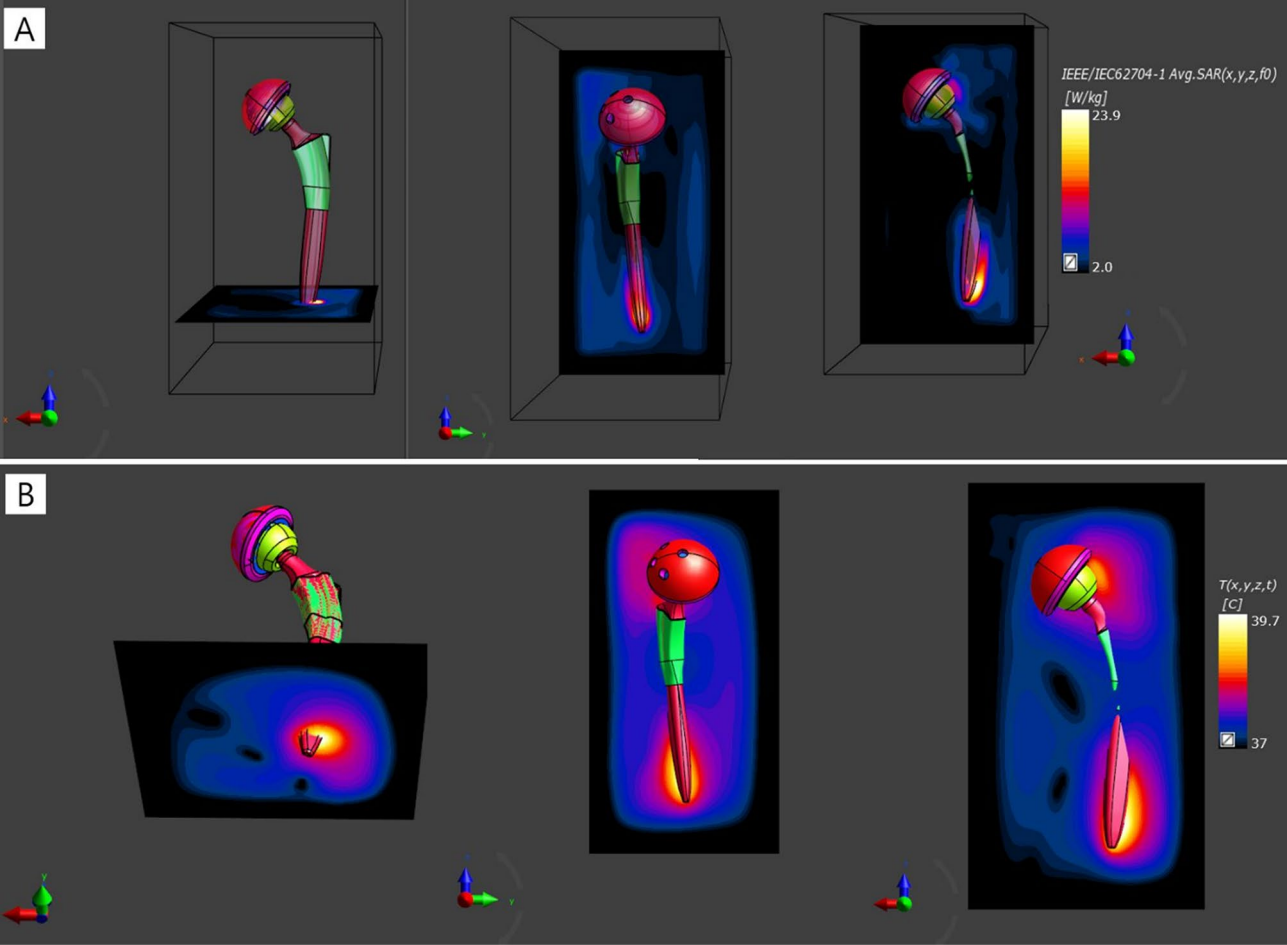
SAR (A) and Temperature distribution (B) near an artificial hip joint using Sim4Life version 4.4 software (Zurich Med Tech, Zurich, Switzerland. https://zmt.swiss/sim4life/). The local SAR values were normalized to the whole-body averaged SAR of 2 W/kg. The SAR value was 23.9 W/kg near the tail of the artificial hip joint. The maximum temperature (= 39.7 °C at 8 min exposure at MRI, compared to base temperature at 37 °C) appear near the tail of the artificial hip joint.
